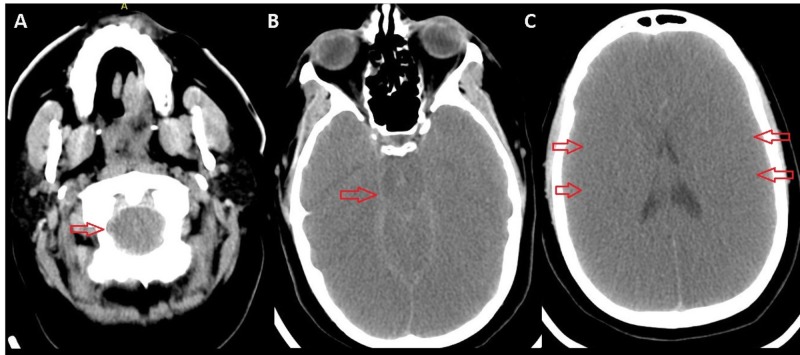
Computed tomography (CT) of the head. Diffuse cerebral edema is noted with tonsillar herniation (A), uncal herniation (B), and loss of cortical sulci (C).
